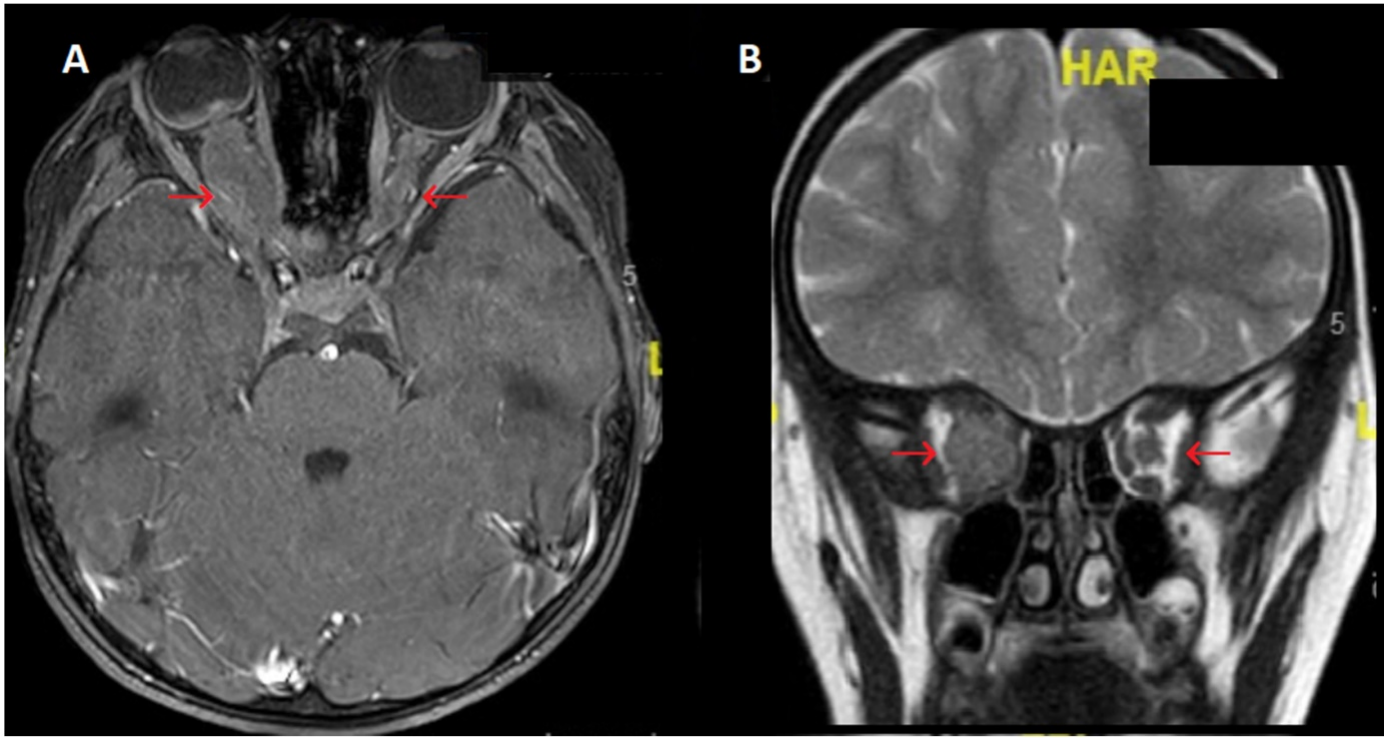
Brain magnetic resonance imaging (MRI) of BCP-ALL ophthalmic relapse for a child with massive infiltration of the right optic nerve. Images show a major circumferential thickening of the homogeneous right optic nerve with little contrast with lesser involvement of the left optic nerve. A. Axial THRIVE sequences after gadolinium injection B. Coronal T2. Legend: ALL: acute lymphoblastic leukemia
